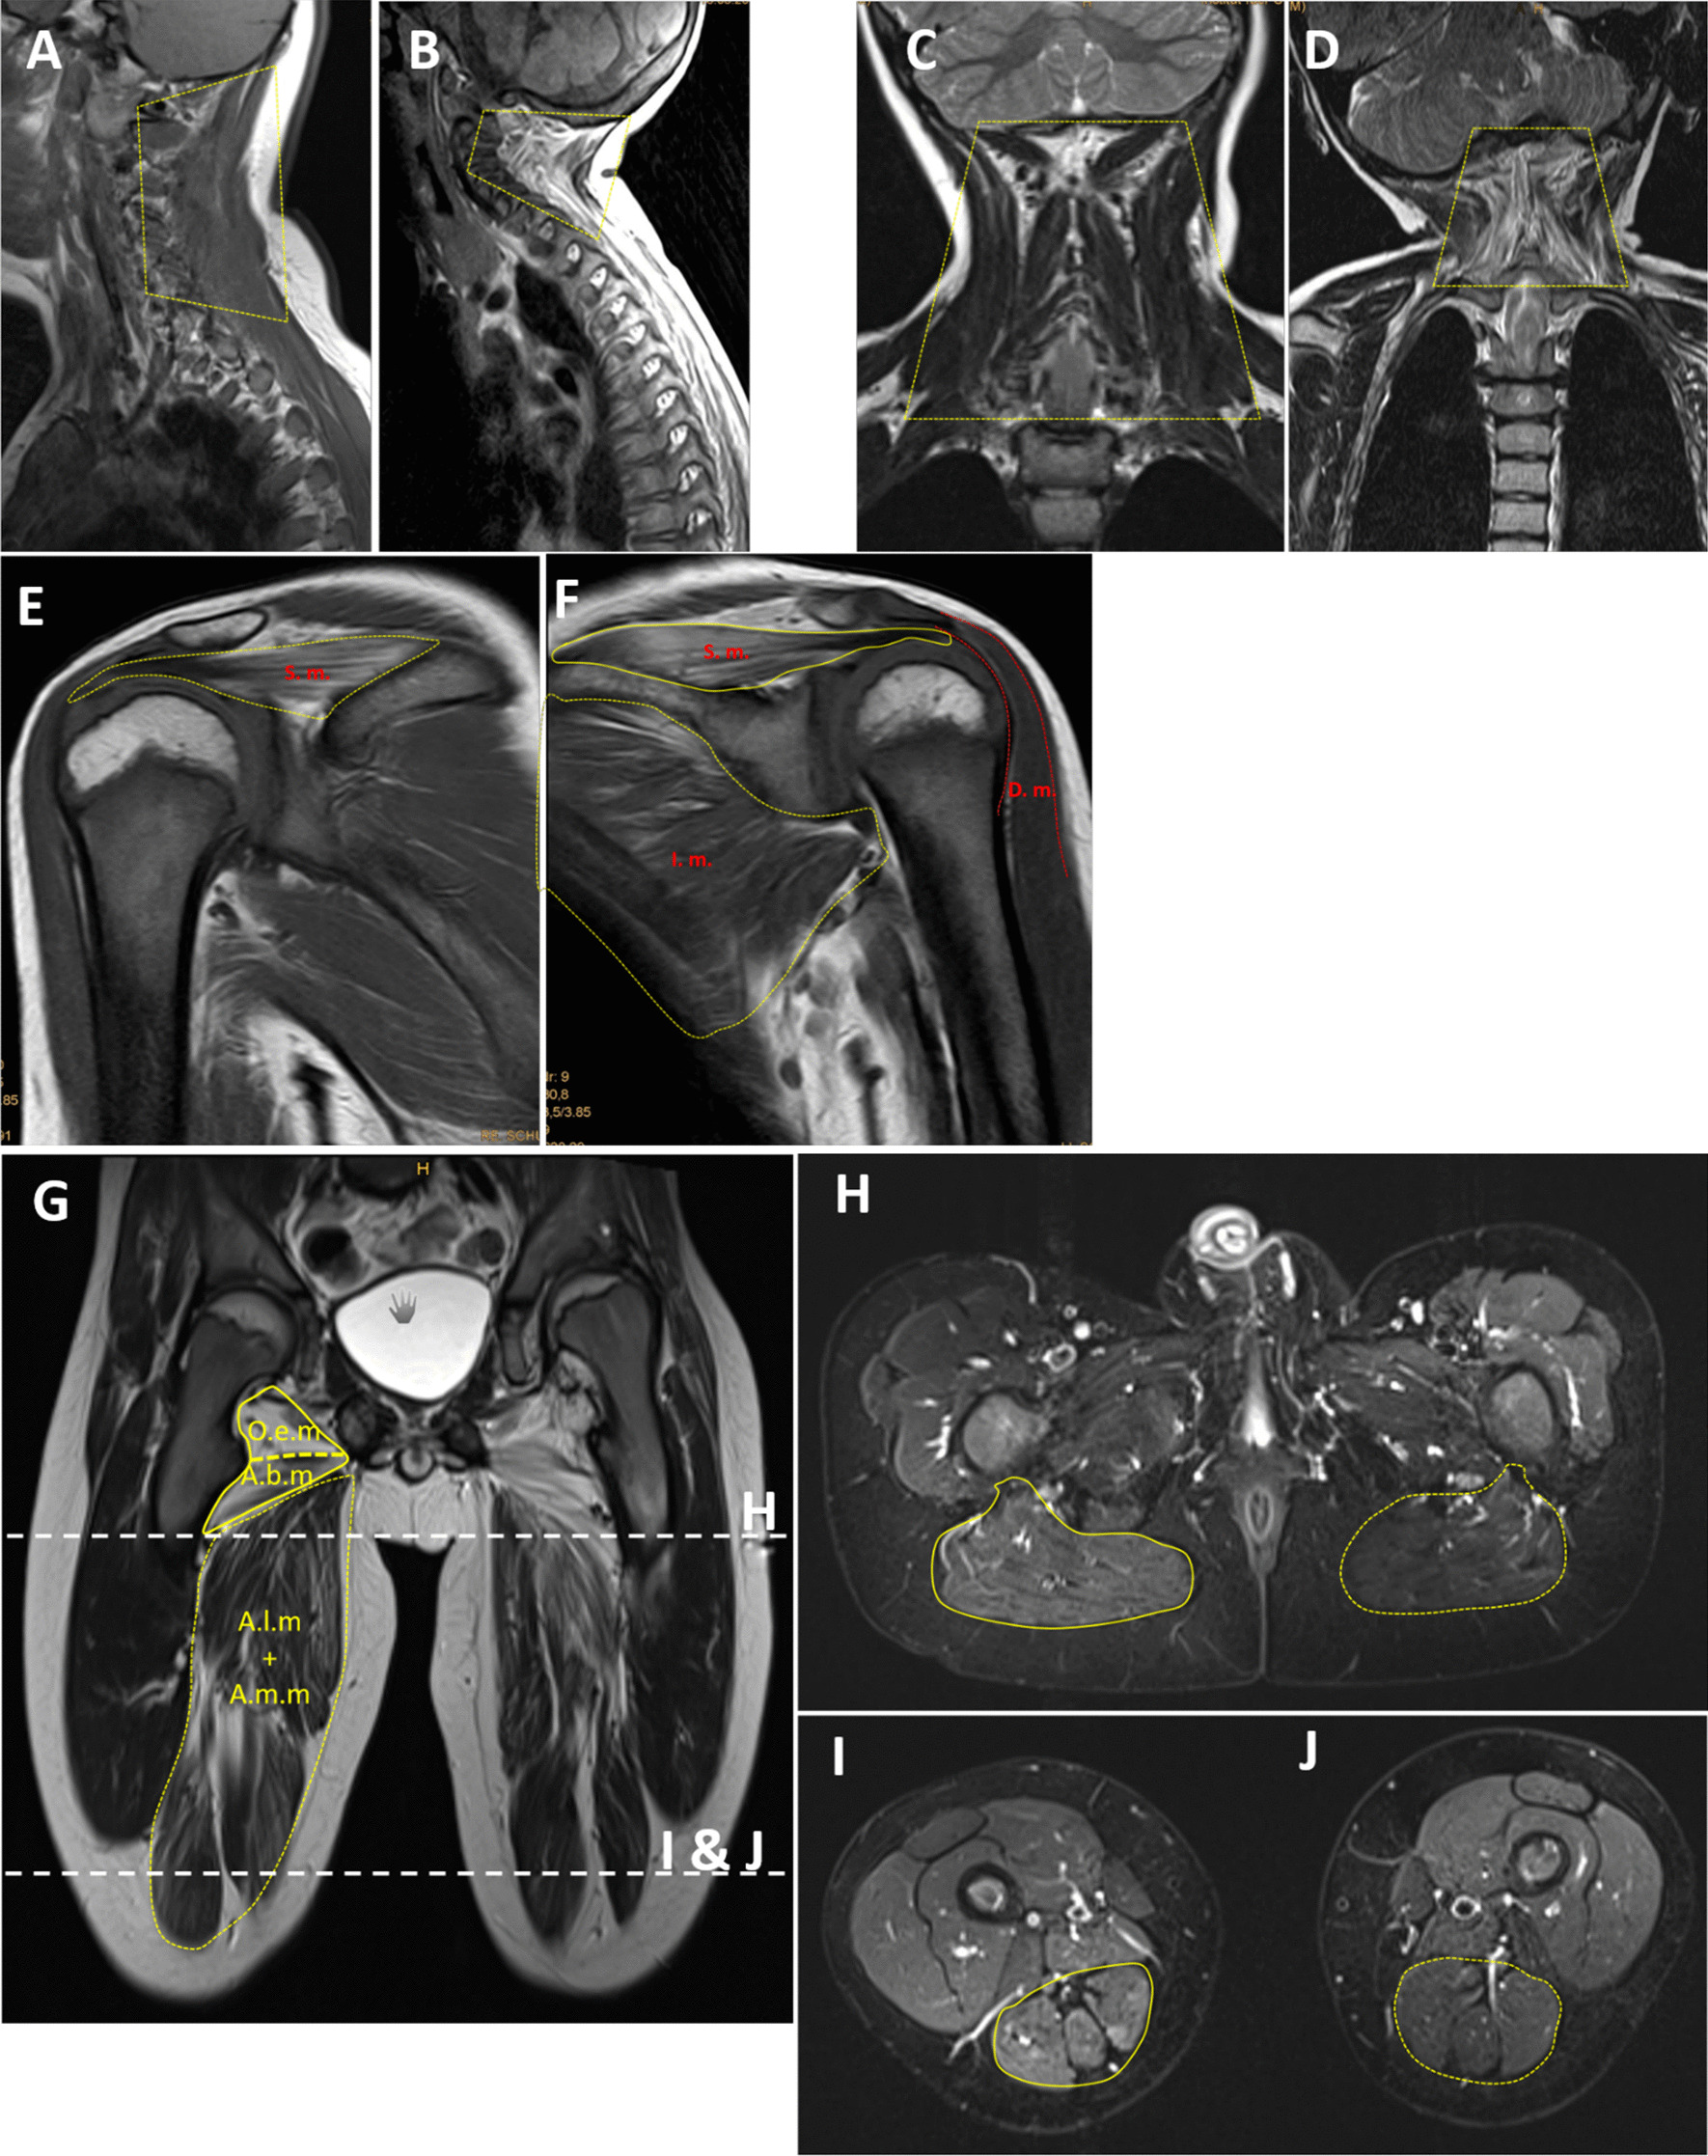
MRI findings of patient 1. Top row, T2 w MRI. (B + D) Sagittal (B) and frontal (D) view of the neck muscles of patient 1 at the age of 7 years. Neck muscles and paravertebral muscles are degenerated with compensatory hyperlordosis of the cervical spine compared to (A + C) age-matched healthy controls. Second row shows the difference between right and left T2 w MRI at the age of 7 years: (E + F) frontal view of right + left shoulder showing symmetrical fatty degeneration of the supraspinatus muscle (S.m.) and involvement of the infraspinatus muscles (I.m.) compared to normal signal for deltoid muscles (D.m. in red). Bottom row shows the difference between right and left T1 w MRI at the age of 9 years: (G) Frontal view of thigh with obturator internus (O.a.m.) and adductor brevis (A.b.m) muscles replaced by fat tissue, adductor longus (A.l.m.) and adductor magnus (A.m.m.) muscles fatty degenerated. (H) A proximal axial view of the hip muscles: with the left gluteus maximus muscle (G.m.m.) showing slight signs of fatty infiltration, the right side appears not to be affected. (I + J) A distal axial view of the thigh muscle showing very slight involvement of the left biceps femoris, sartorius and semimembranosus muscles (circled in yellow) and no obvious involvement of the right thigh
